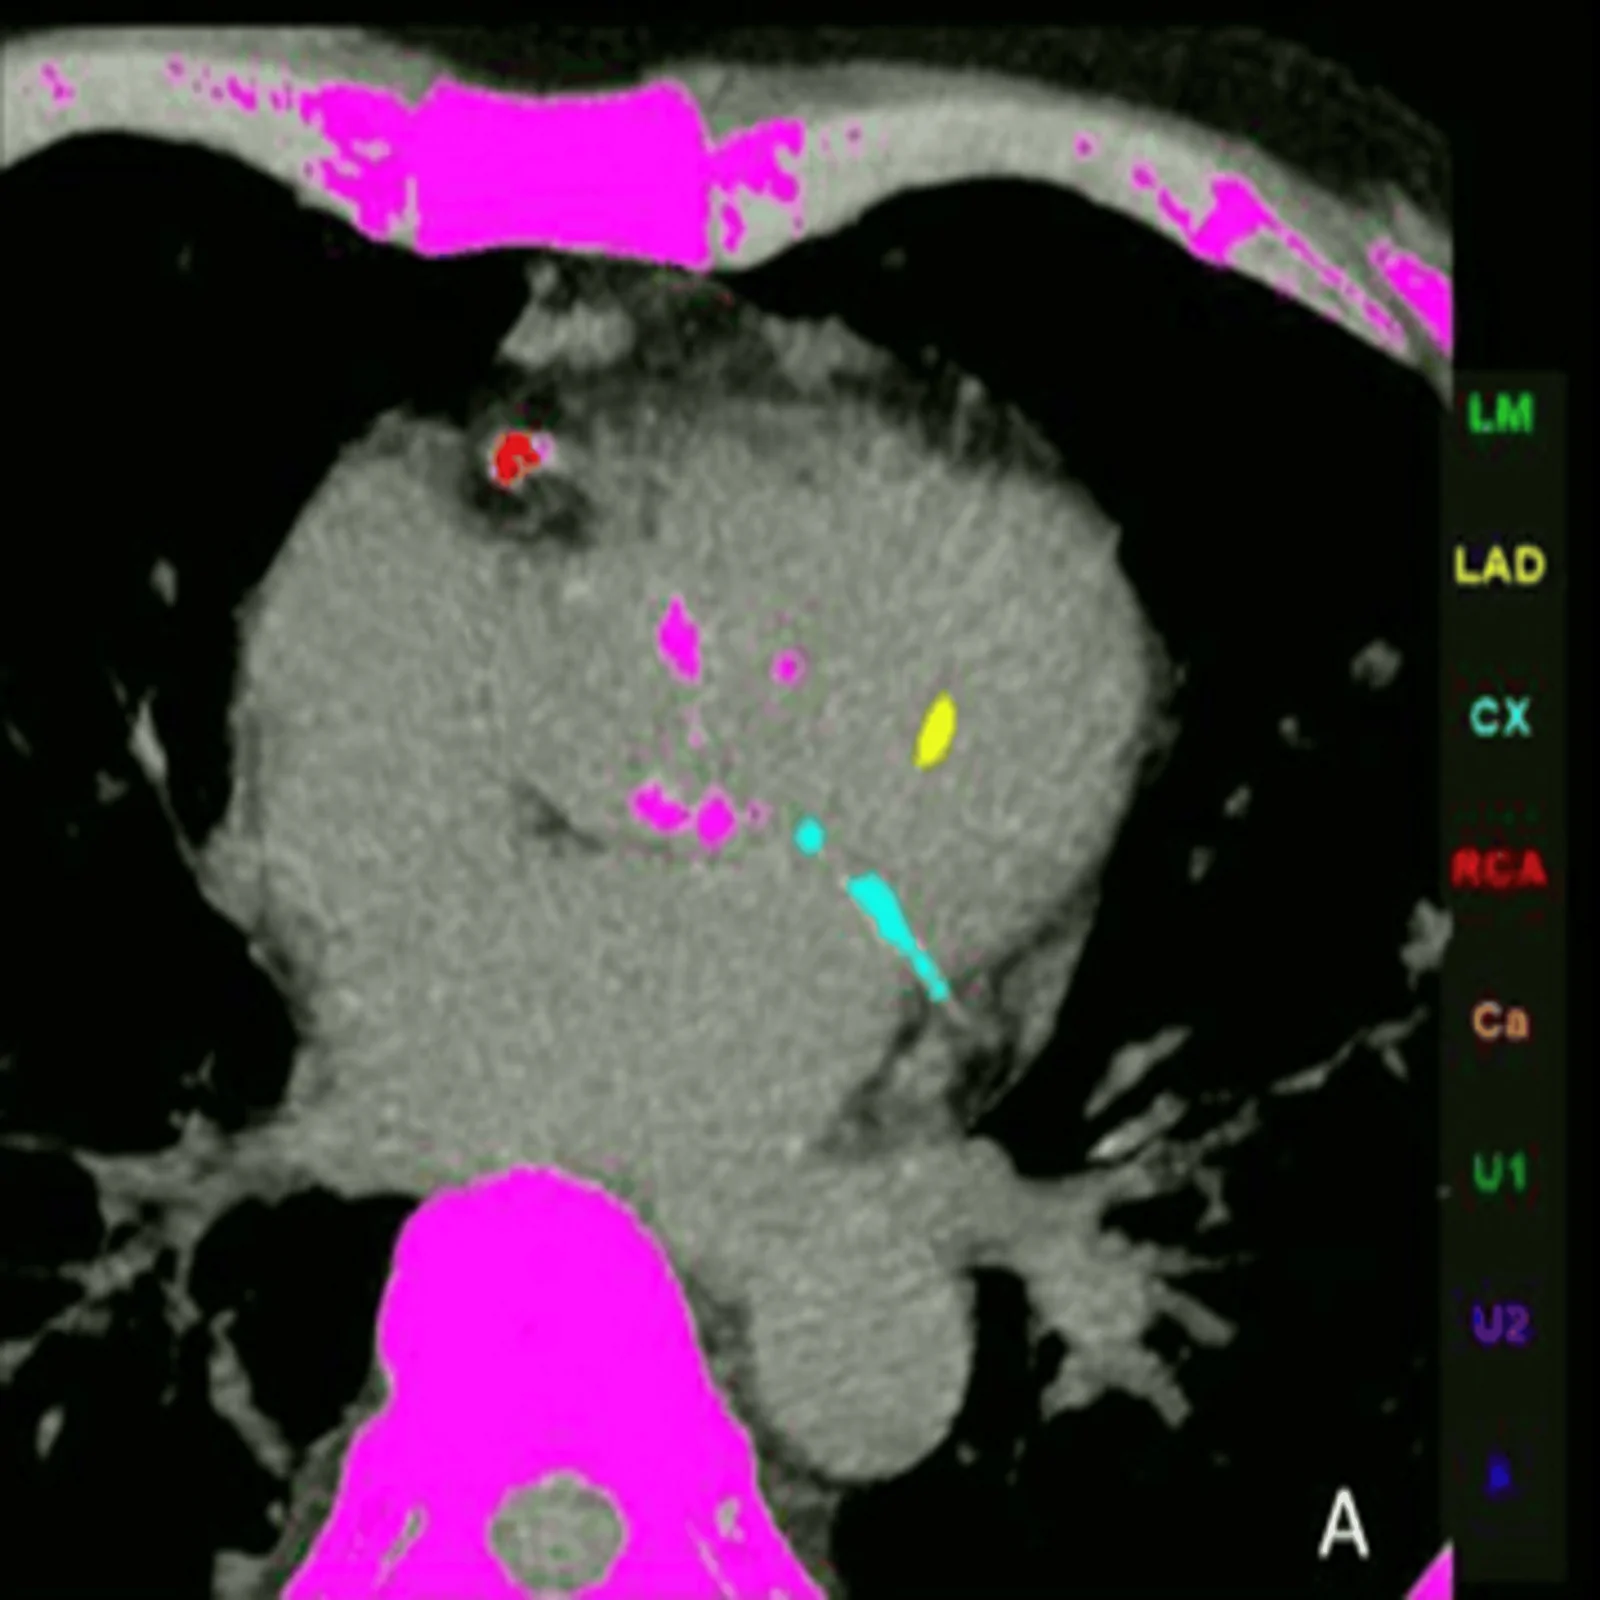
Axial non-contrast cardiac computed tomography demonstrating color-coded calcified plaques in the left anterior descending artery (yellow), left circumflex artery (cyan), and right coronary artery (red) used for Agatston scoring.
